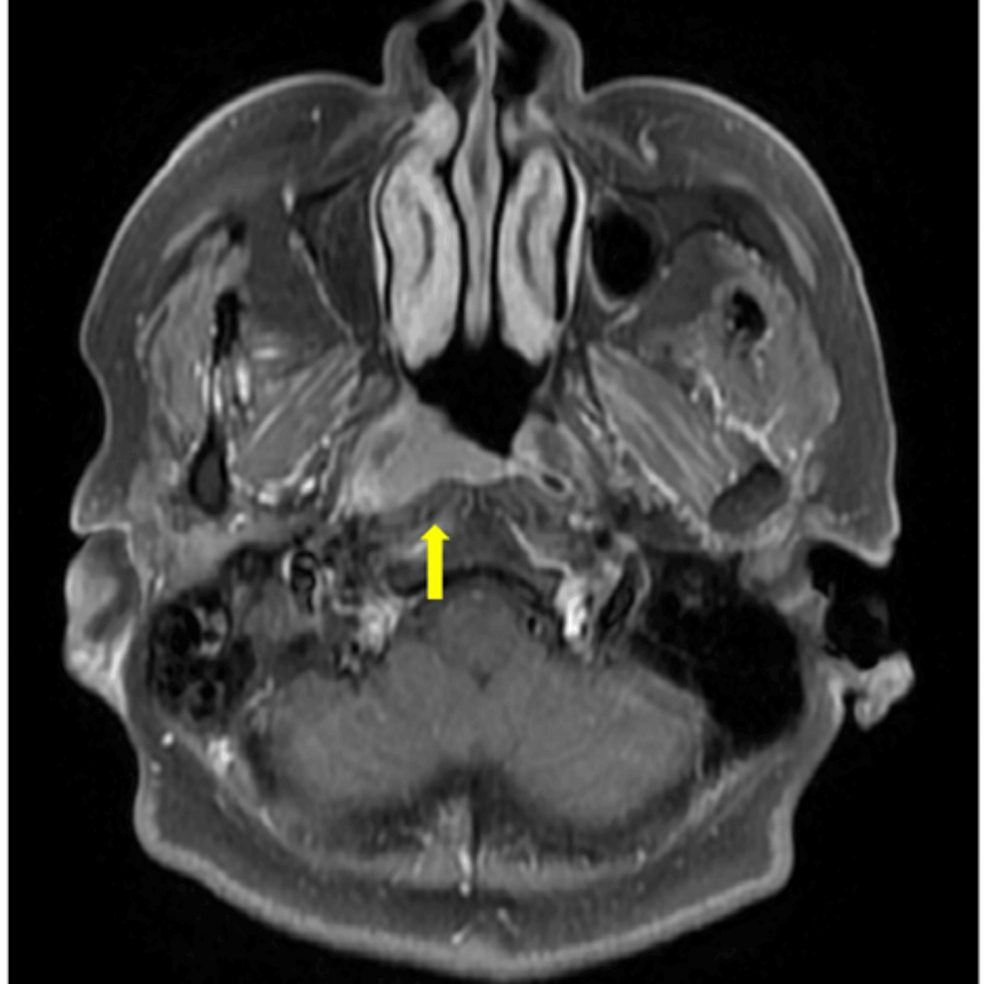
Magnetic resonance imaging before the first treatment The tumor (arrow) was diagnosed as cT1.
